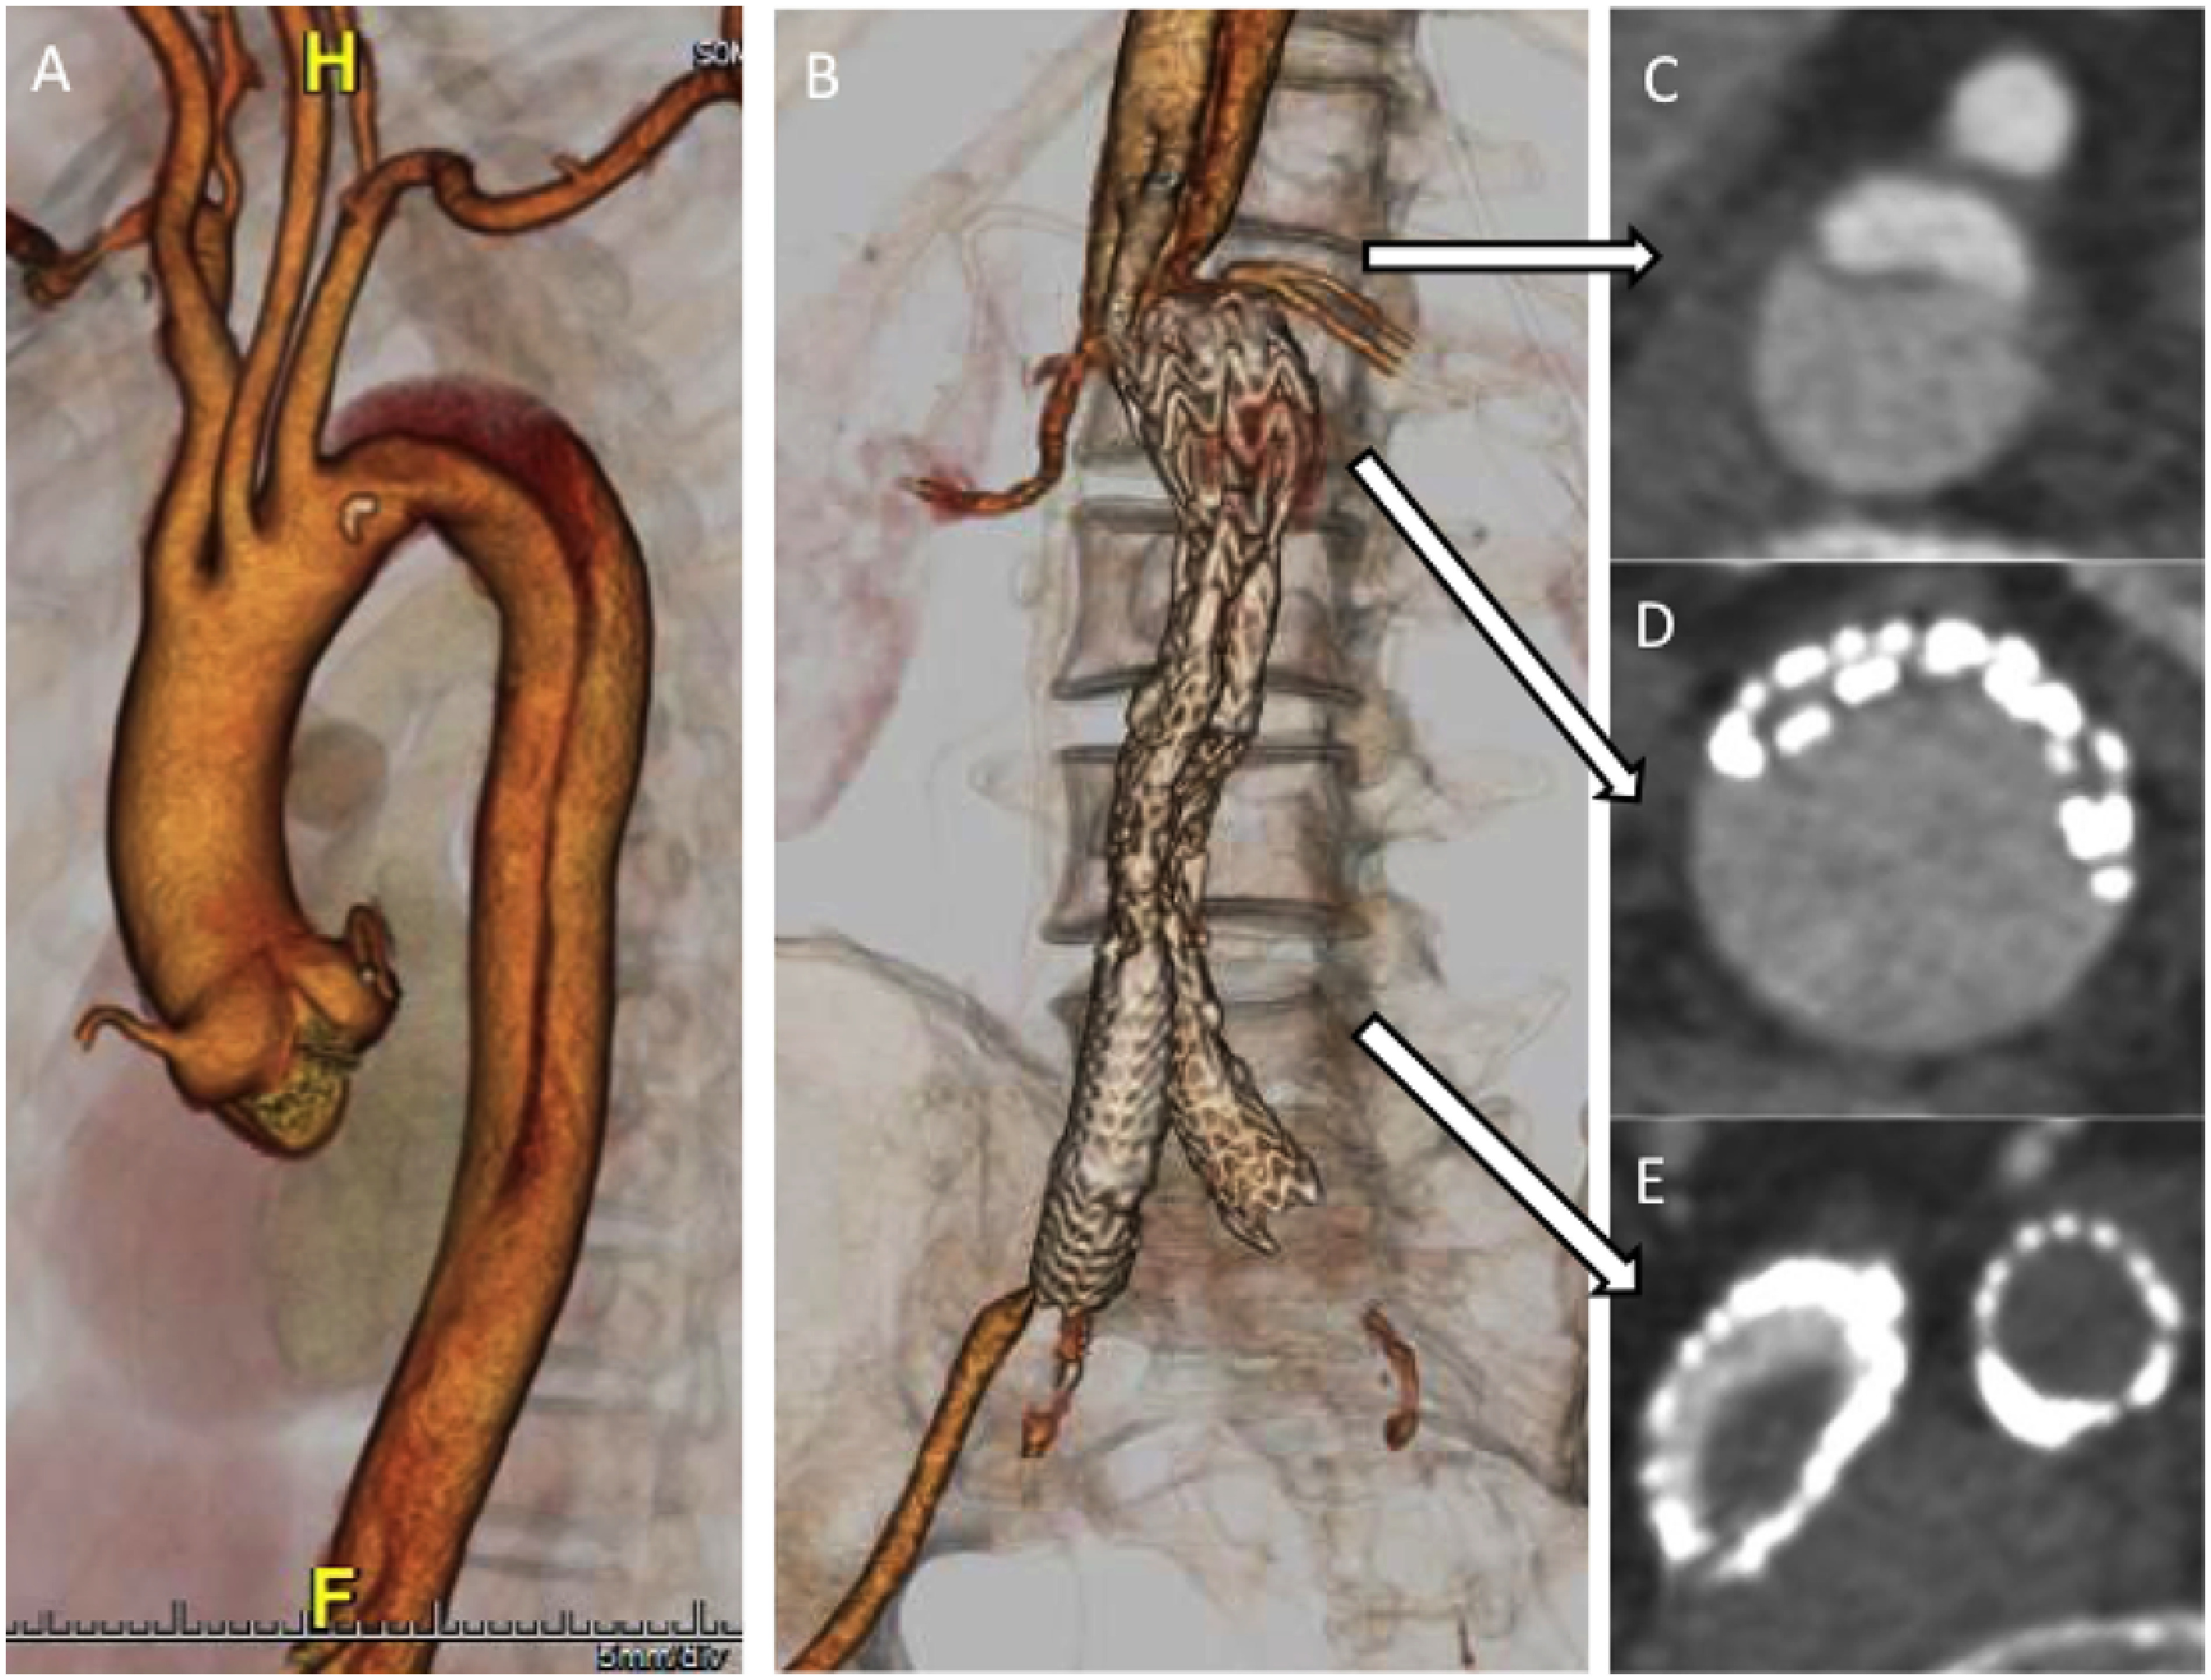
Fig. 2 (A), (B) At the onset of acute type B aortic dissection, CT scan showed the dissection extended from the descending aorta to the bilateral common iliac artery. Aortic dissection progressed retrogradely to the descending aorta. There was no obvious re-entry. The maximum short diameter of the aneurysm is 47 mm and the false lumen diameter is 37 mm, which imply a high value of the false lumen pressure. (C) The entry was observed near the superior mesenteric artery origin. (D) The stent graft was compressed and completely occluded by the false lumen pressure. (E) The blood flow to the left leg is completely interrupted.
